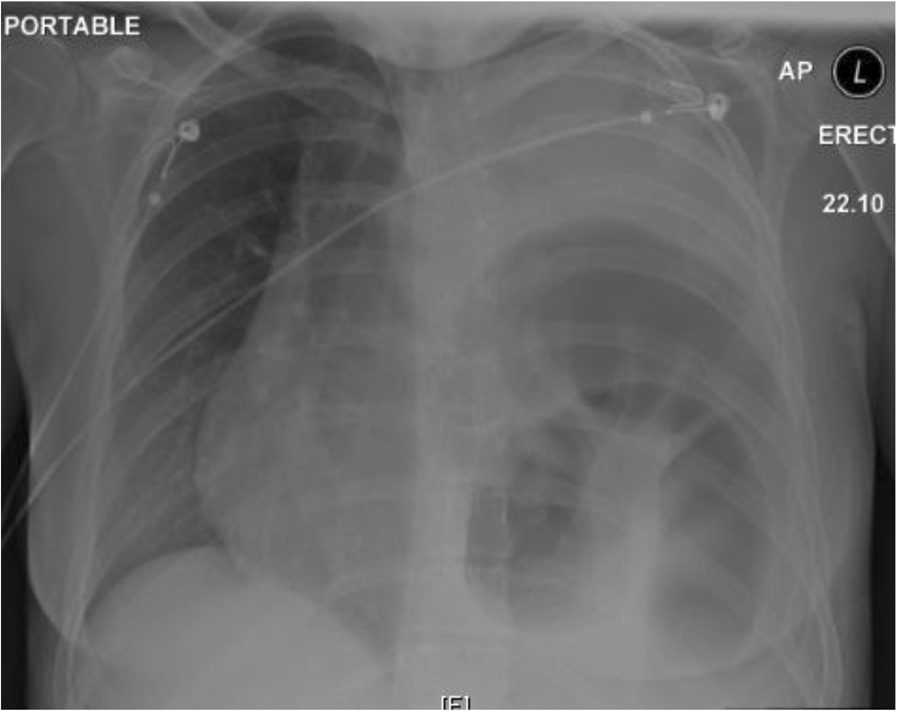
The Chest-X-Ray performed after laparoscopic surgery. It shows dilated bowels loops in the left hemi thorax shifting the mediastinum to the right.
